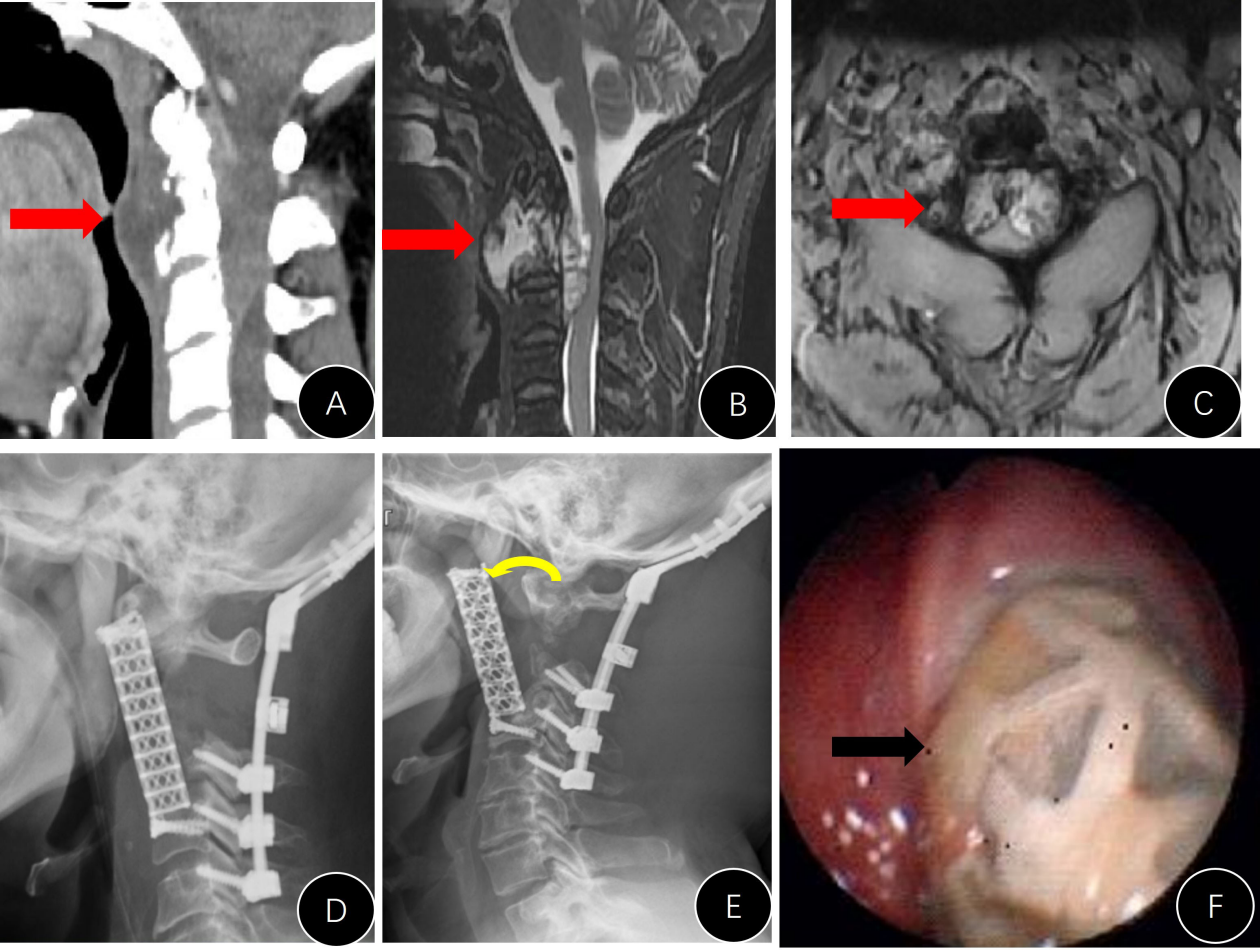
Presentation of #A9. (A–C) Preoperative CT and MRI images; (D) postoperative lateral x-ray; (E) lateral x-ray 3 years after the operation (anterior rotation of the titanium mesh); (F) unhealing of the pharyngeal wall with mesh exposure.
